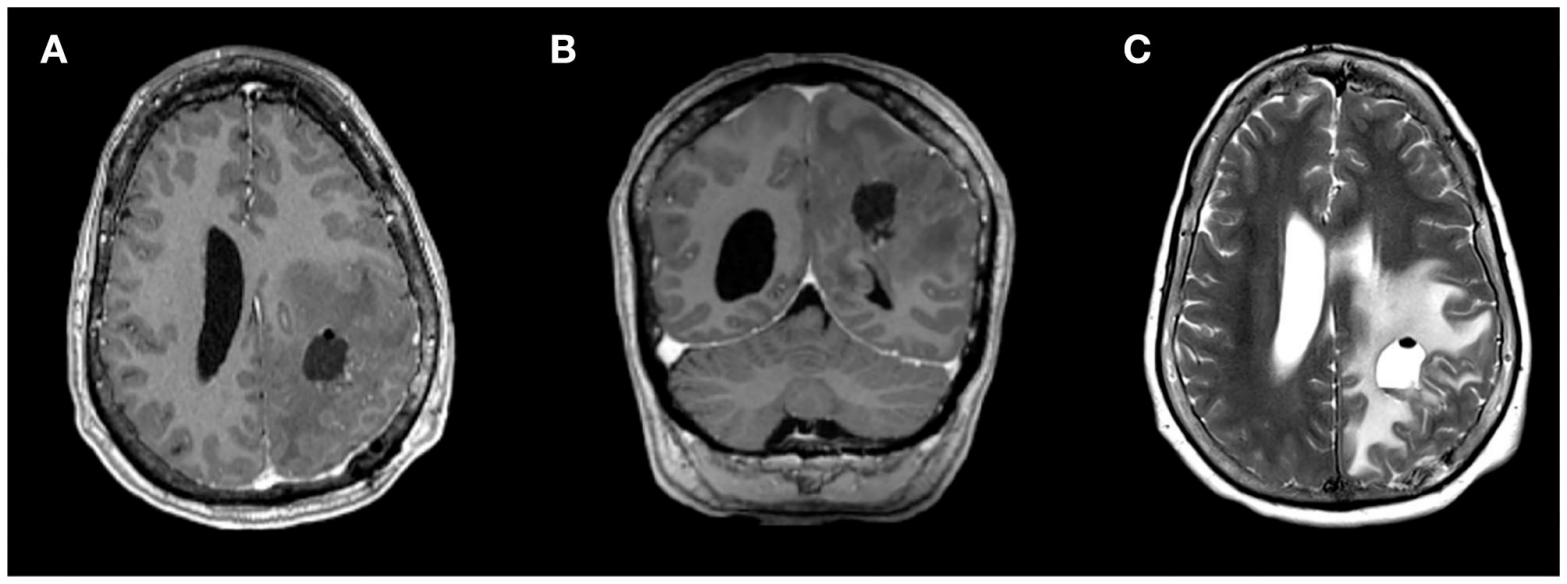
Magnetic resonance imaging (MRI) from post-operative day 1 showing gross total resection of the dominant left frontoparietal mass, on (A) axial and (B) coronal contrast-enhanced T1-weighted sequences and (C) T2-weighted sequence.
